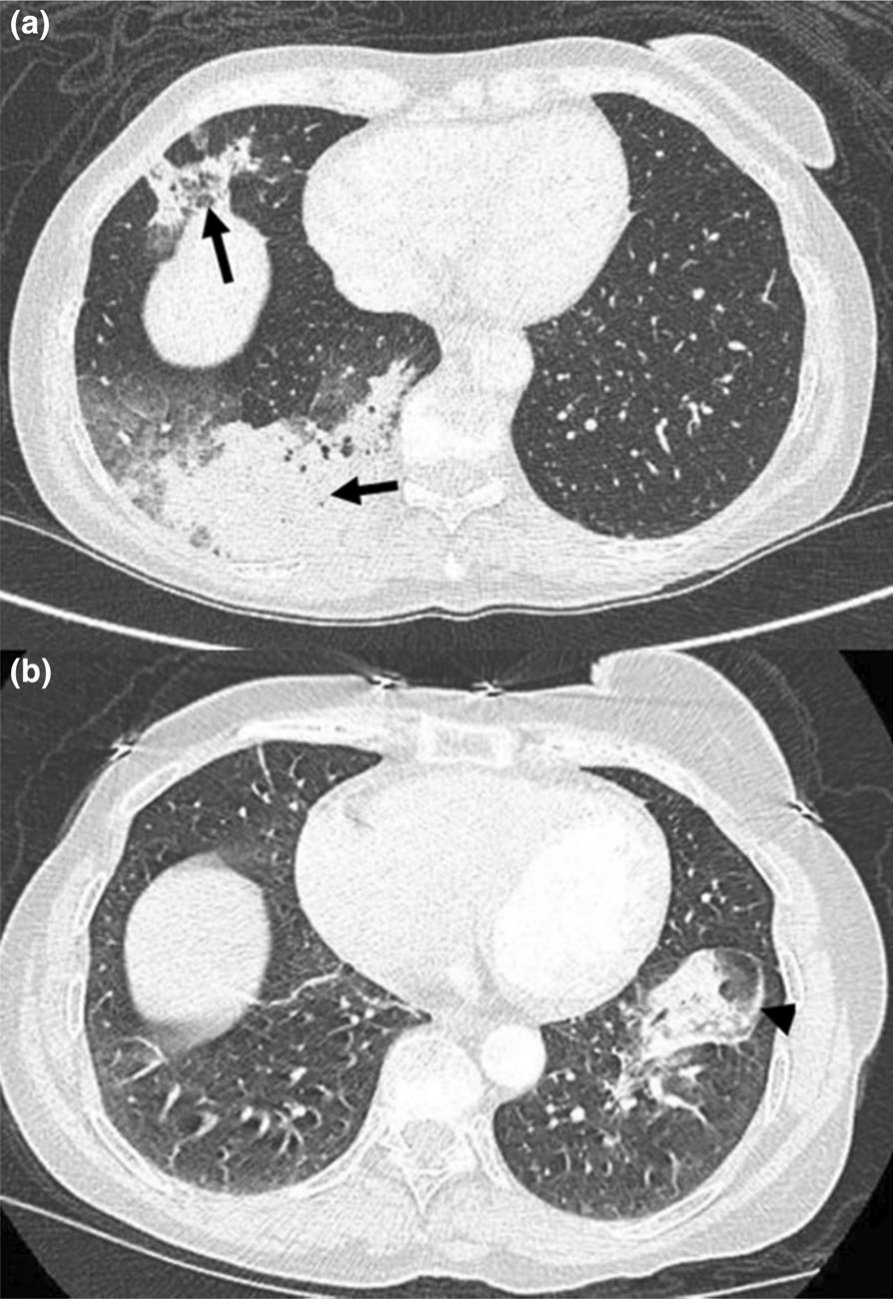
Axial CT chest in a 53‐year‐old female patient with GPA demonstrating confluent alveolar densities in the right middle and lower lobes (arrows) (a), which resolved 6 months later (b). However, new airspace density developed in the left lower lobe (arrowhead) (b), which would be consistent with a migratory pattern.
